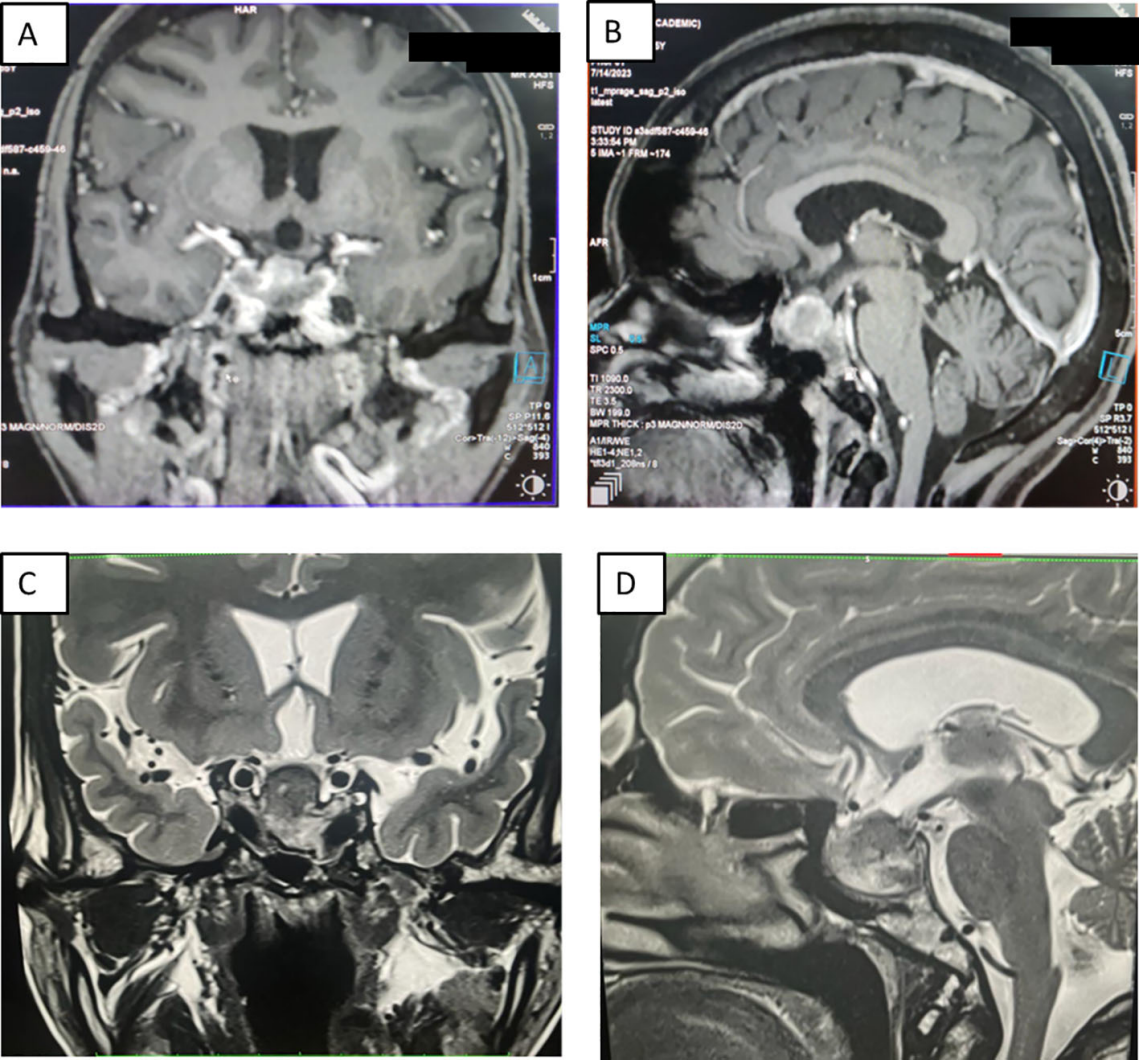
Panel of a contrast-enhanced MRI of the sella showing the initial reduction in size of a residual lesion to 20×24×24 mm (48% reduction) 3 months after lung surgery on coronal (A) and sagittal sections (B). Further significant reduction of the lesion to 19×20×17.6 mm (70% reduction) in the coronal (C) and sagittal (D) sections 9 months after lung surgery.
